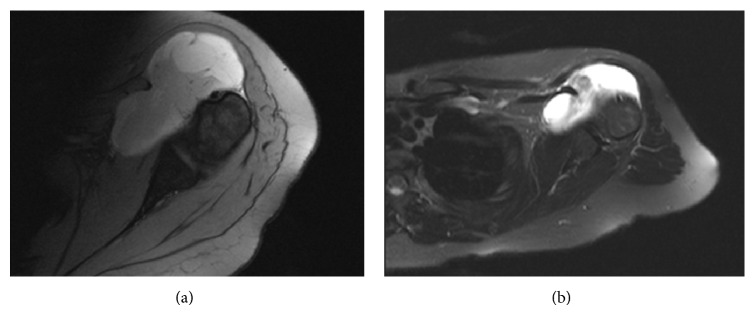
(a) Pre- and (b) postradiation therapy MRI of synoviosarcoma of the anterior shoulder joint.
